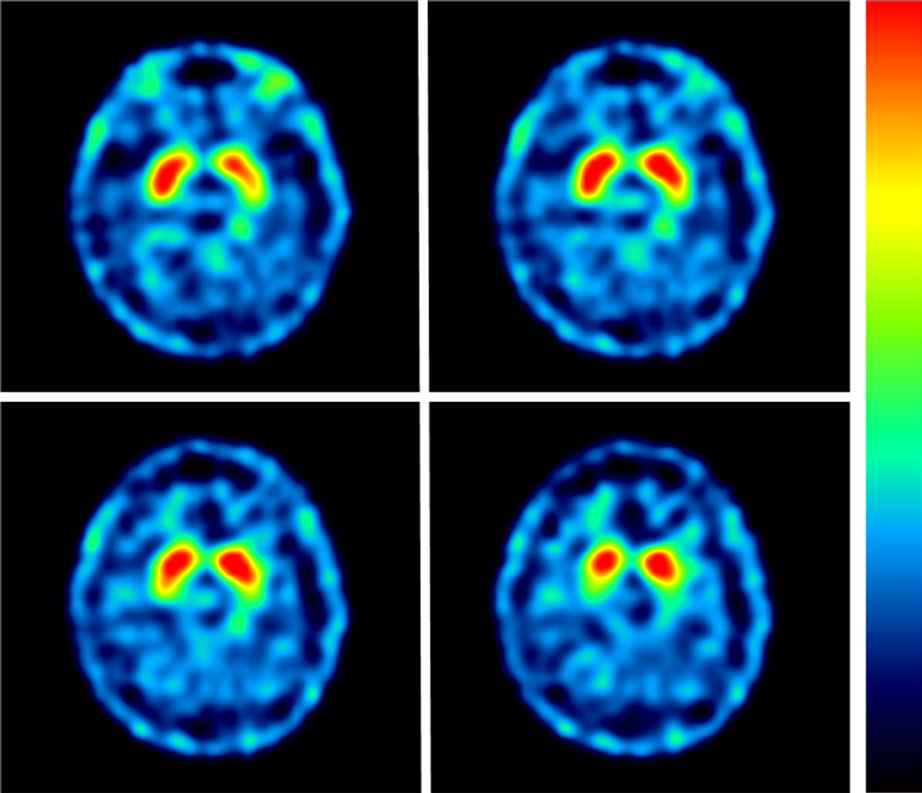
Image of 99mTc‐TRODAT‐1 SPECT of index patient. The image revealed bilateral symmetry of the normally shaped caudate nucleus and putamen. Symmetrical distribution of dopaminergic radioactivity was noted in the striatum. The result revealed no presynaptic uptake decreasing
